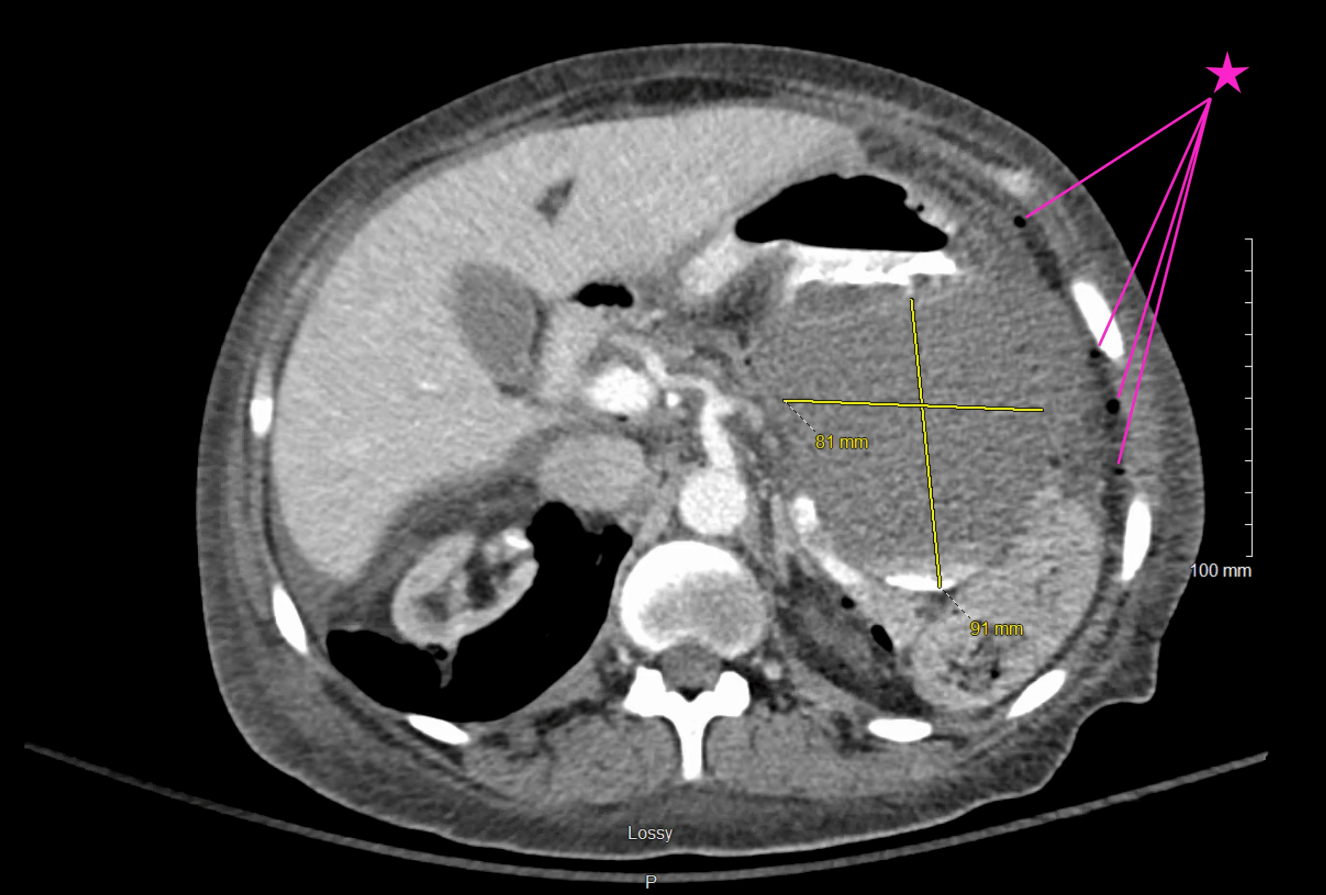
CT imaging reveals the sequelae of a bowel perforation including adjacent fluid collection (indicated by yellow measured cross) with dependent contrast pooling. The pink star points to several small pockets of free air CT: computed tomography
